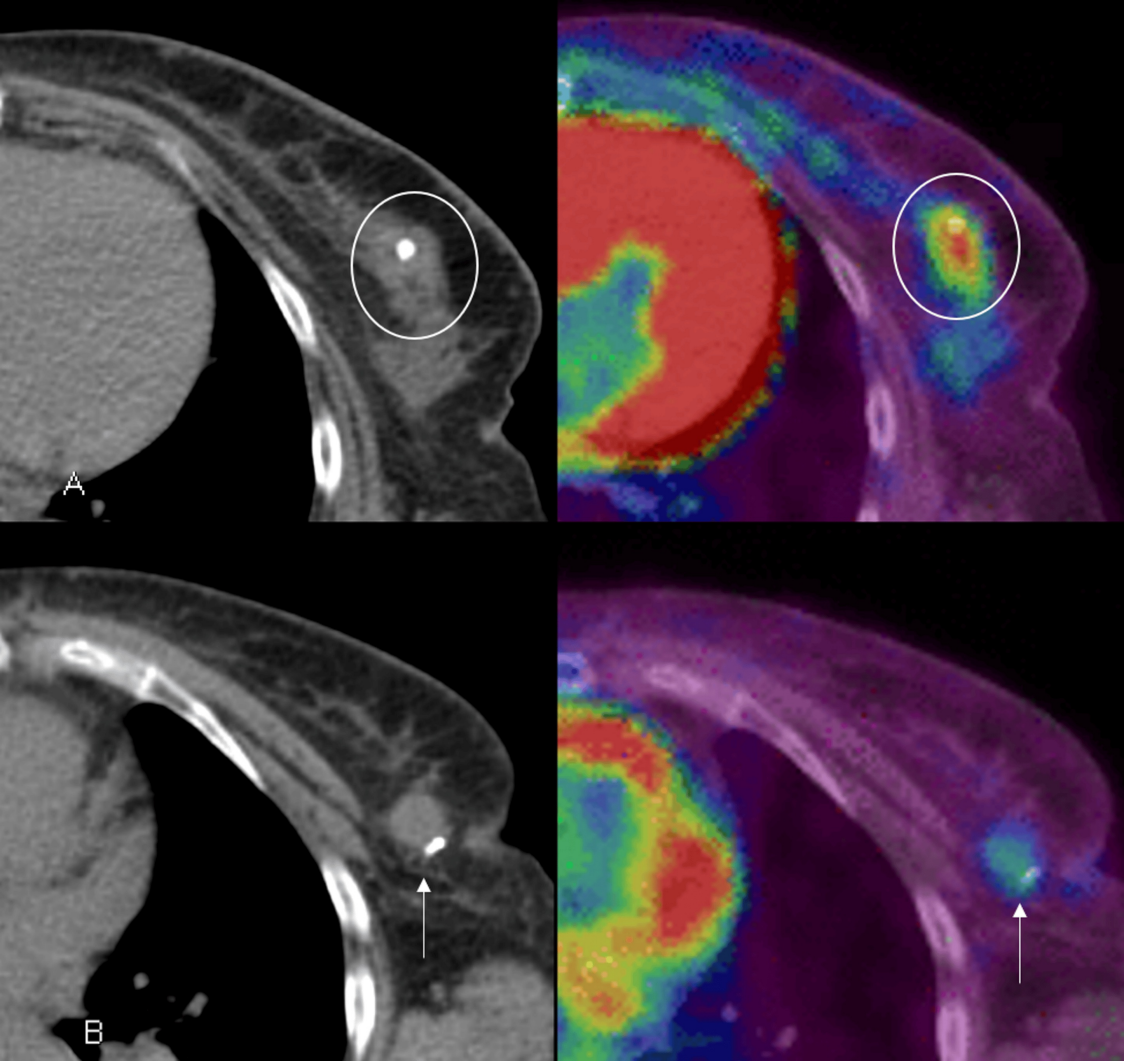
PET/CT PET/CT for the extent of the disease was performed one month after diagnosis and demonstrates the biopsy proven malignancy (A, circles), demonstrating intense radiotracer uptake (right image), and the pseudoaneurysm, seen as a soft tissue density (B, arrows), demonstrating mild radiotracer uptake (right image).
